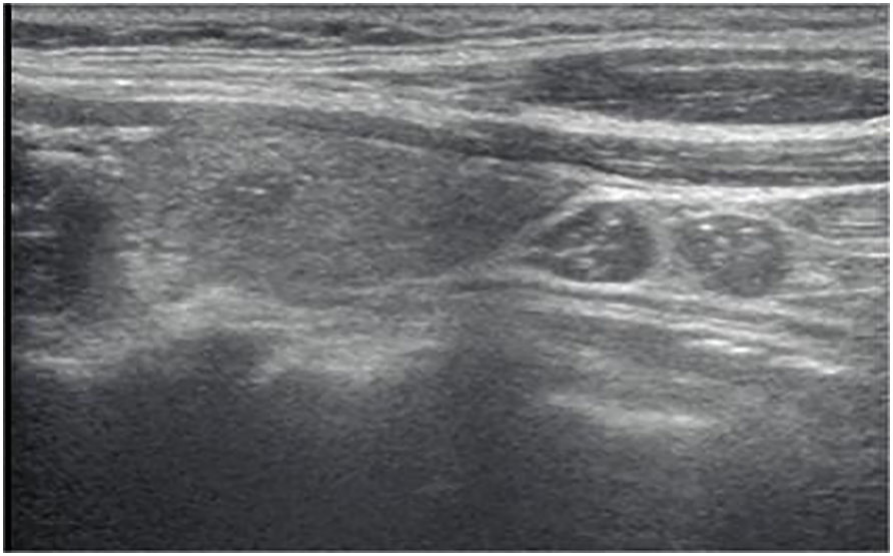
Longitudinal ultrasonography image from a 39-year-old woman with papillary thyroid carcinoma shows a 6-mm solid, hypoechoic, and ill-defined margin thyroid nodule with punctate echogenic foci, associated with LN metastases presenting as diffuse hyperechogenicity with punctate echogenic foci, L/S<2.The nodule was classified as EU-TIRADS 5:high risk or K-TIRADS 5:high suspicion. The LNs in level VI were classified as “suspicious for malignancy” according to the EU-TIRADS category and “suspicious” for the K-TIRADS category. The FNA failed based on nodule size, while according to the LNs was suggested.
